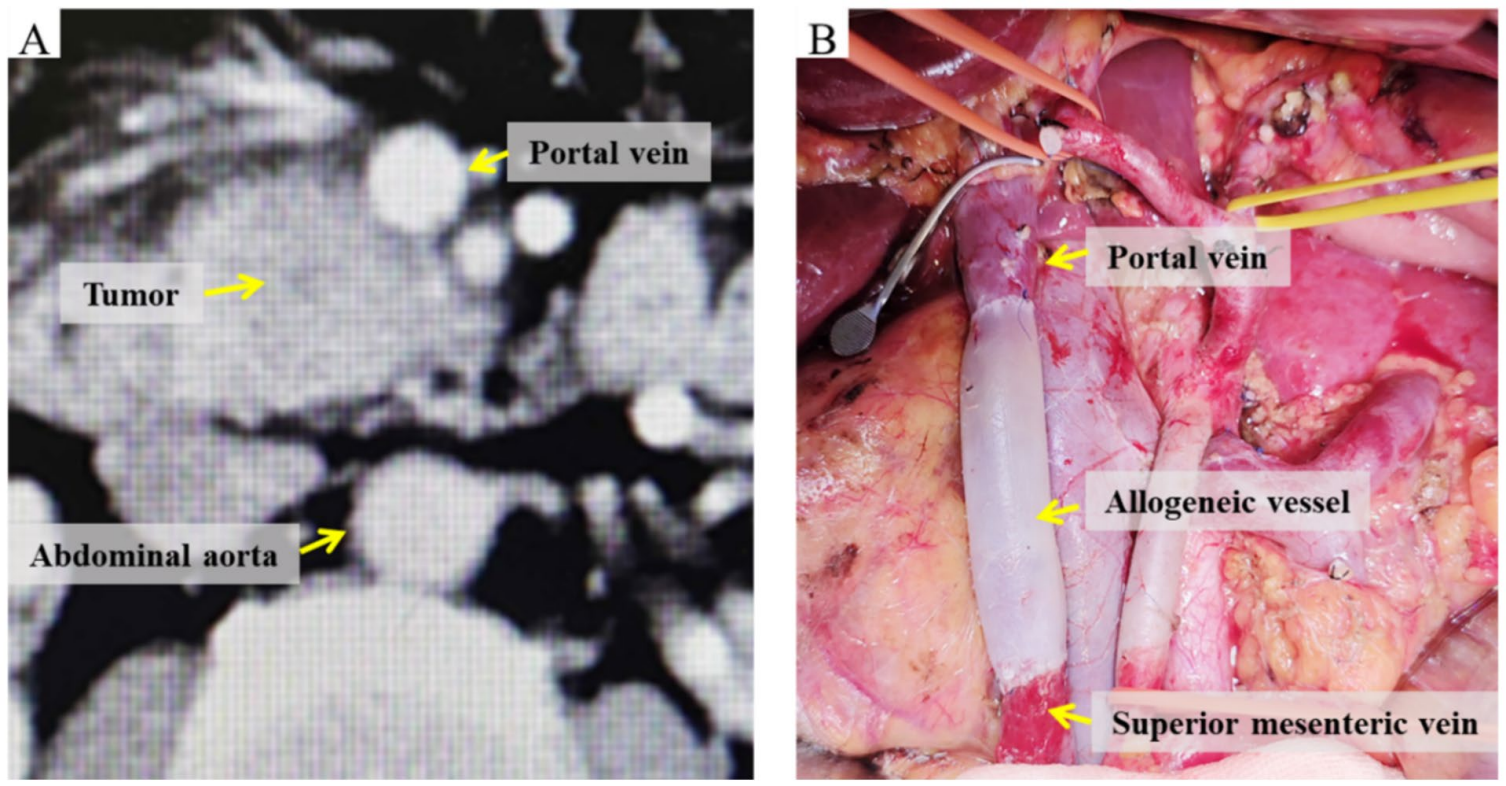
Images of the involved vein before and after radical PD with venous resection and reconstruction. (A) Preoperative CT demonstrating tumor invasion of the portal vein. (B) Intraoperative view of allogeneic vein graft reconstruction.
